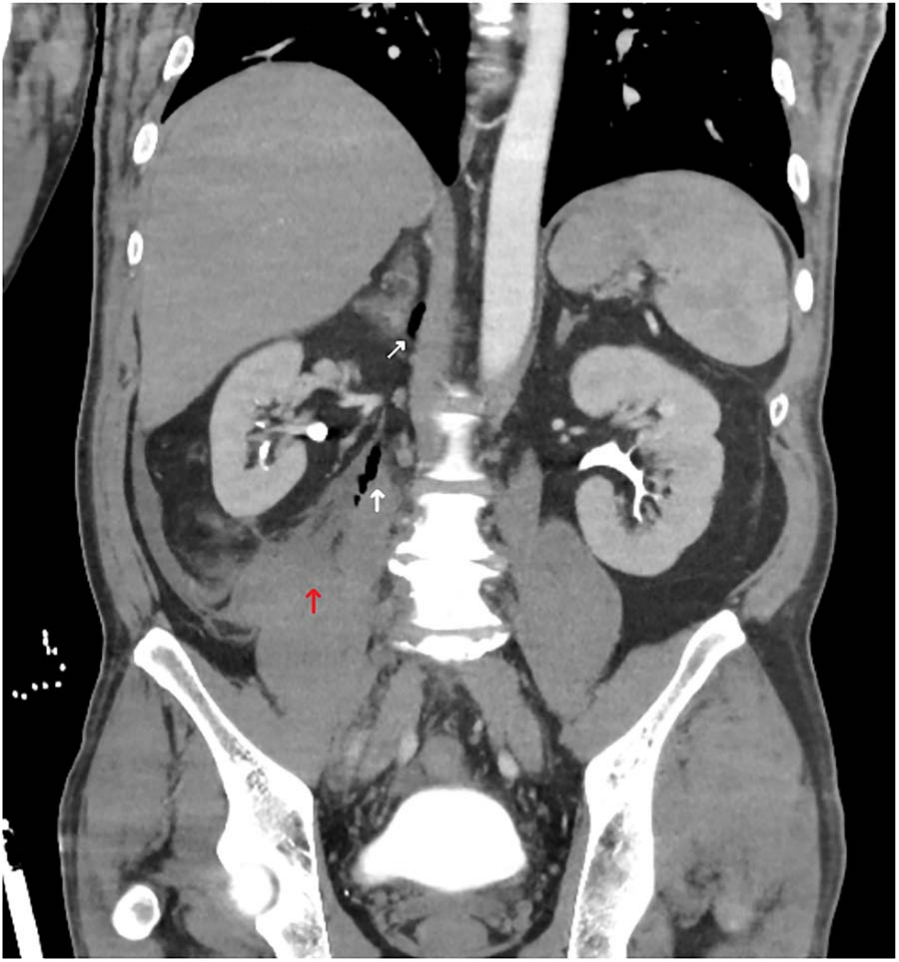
Abdominal CT coronal slice demonstrating a right-sided retroperitoneal haematoma with gas locules tracking up towards the right adrenal.
